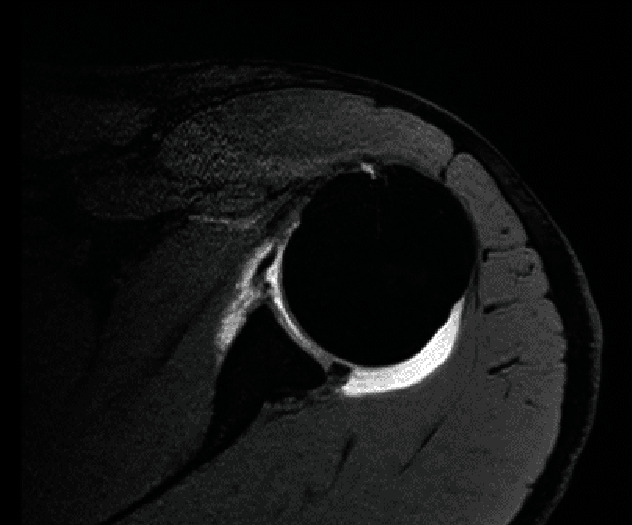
Magnetic resonance imaging axial view demonstrating disruption of right latissimus dorsi tendon.
